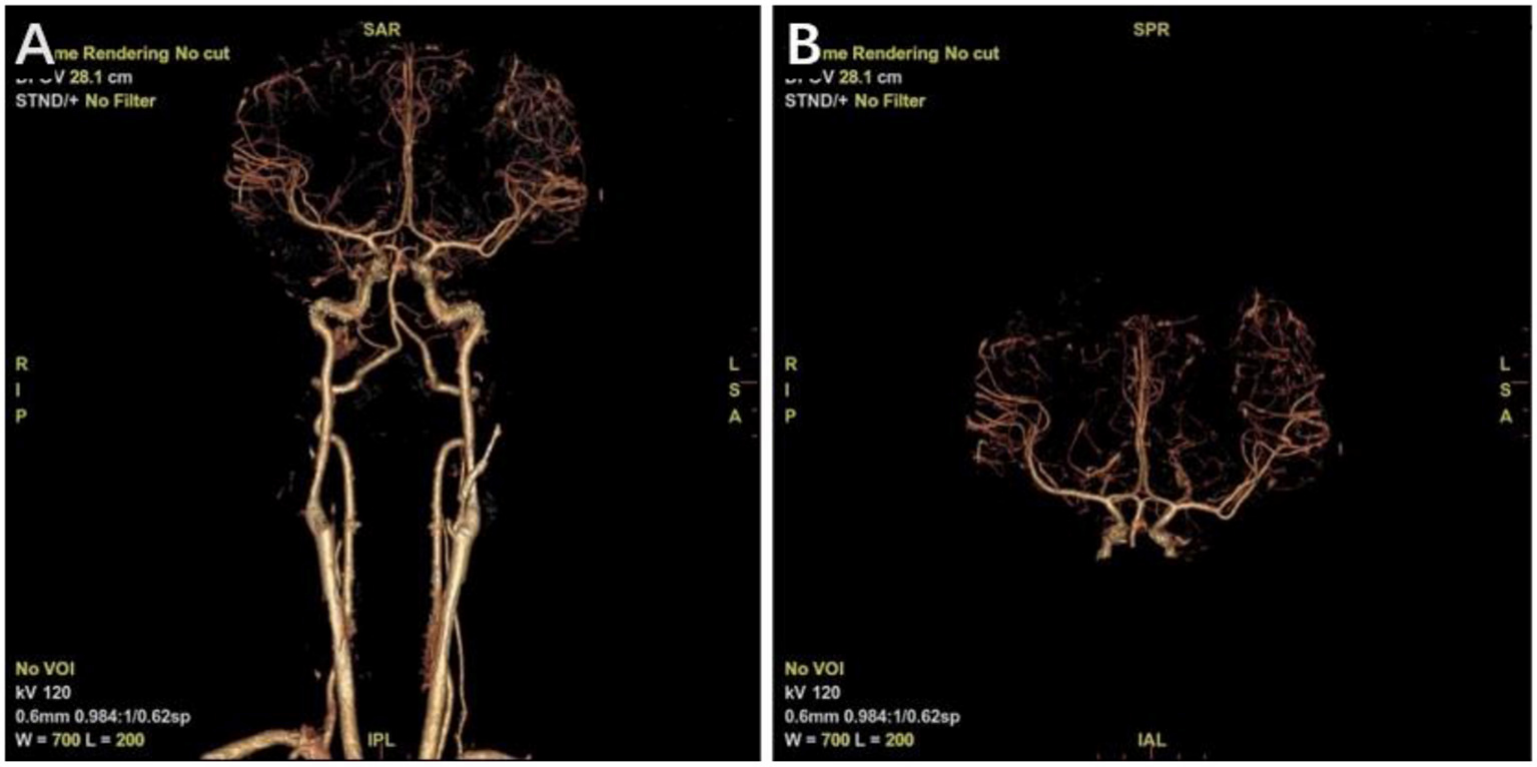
Computed tomography angiography (CTA) at 6 months postoperatively. (A) Whole figure. (B) Local amplification.
